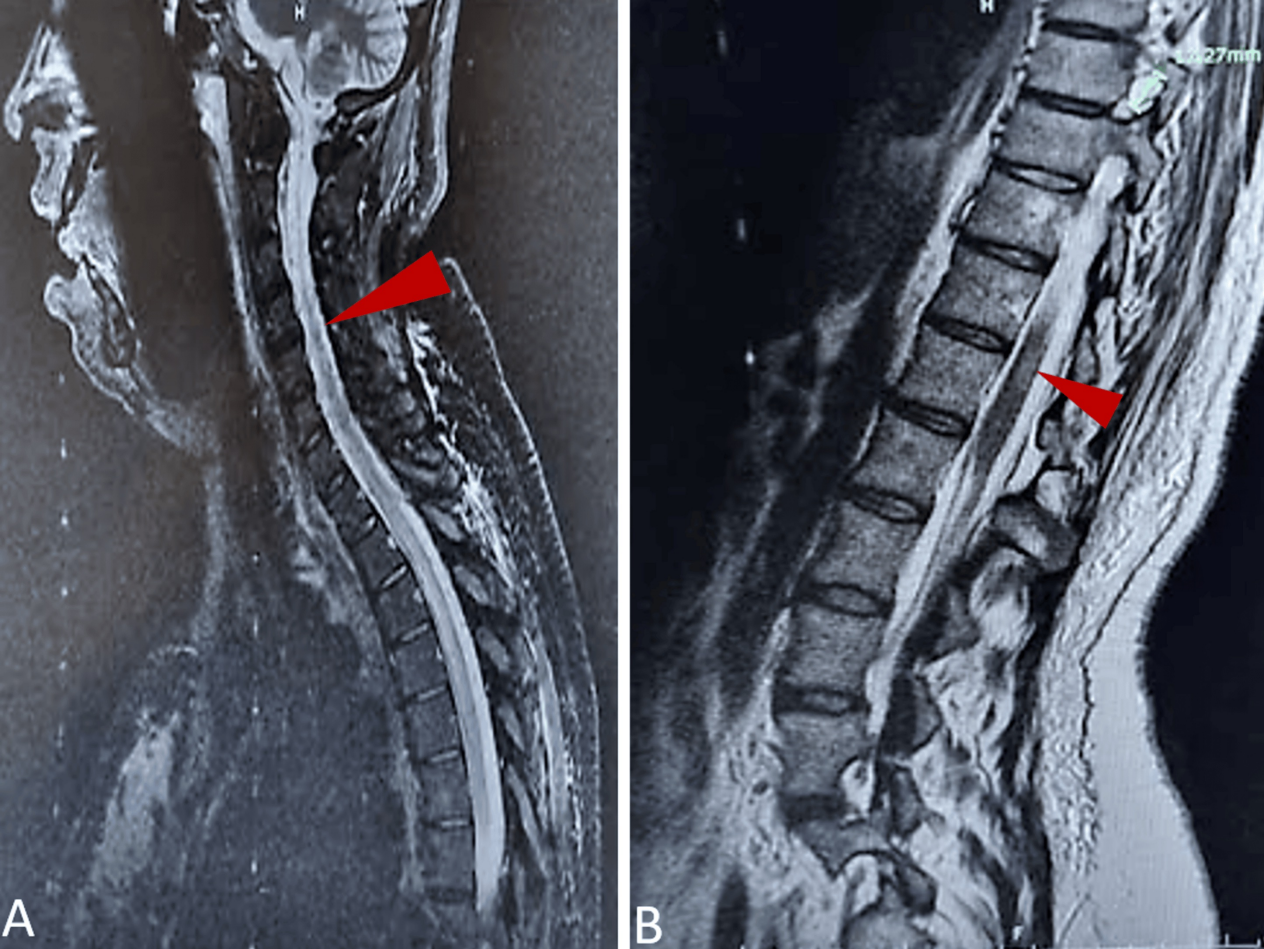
Sagittal section MRI of the spine showing centromedullary edema in the lower cervical region (arrow head) (A). Sagittal section MRI of the spine showing centromedullary edema extending to the conus medullaris (arrow head) (B).
